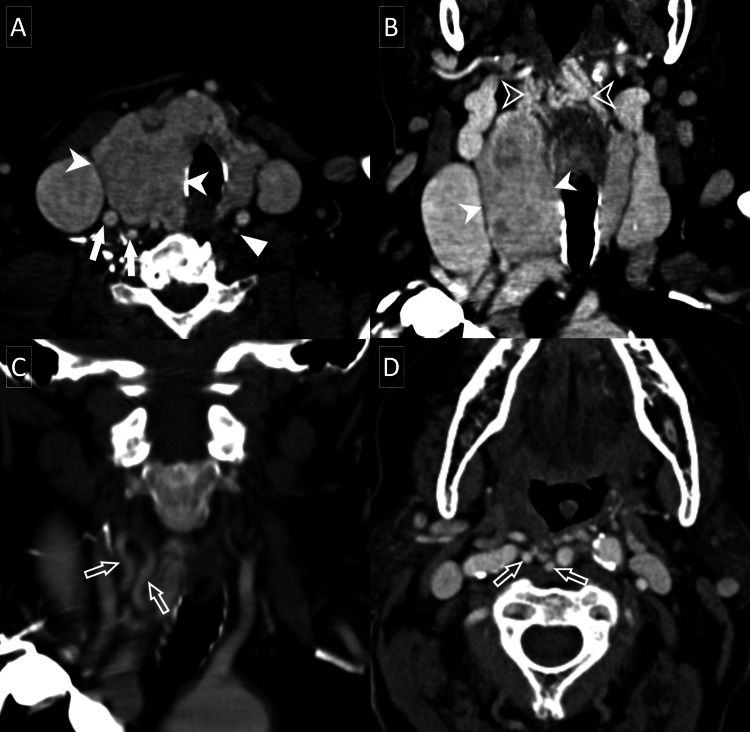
CT of the soft tissue neck with intravenous contrast axial (A, D) and coronal (B, C) views. The most recent CT of the soft tissue neck with intravenous contrast demonstrates an enlarged heterogeneously enhancing thyroid with an exophytic right thyroid lobe nodule (solid arrowheads) extending into the isthmus. The nodule is supplied by enlarged and tortuous inferior (solid arrows) and superior (hollow arrows) thyroidal arteries. Extensive arterial and venous collaterals are noted (hollow arrowheads). The normal right inferior thyroid artery is denoted by a solid triangle for comparison.
